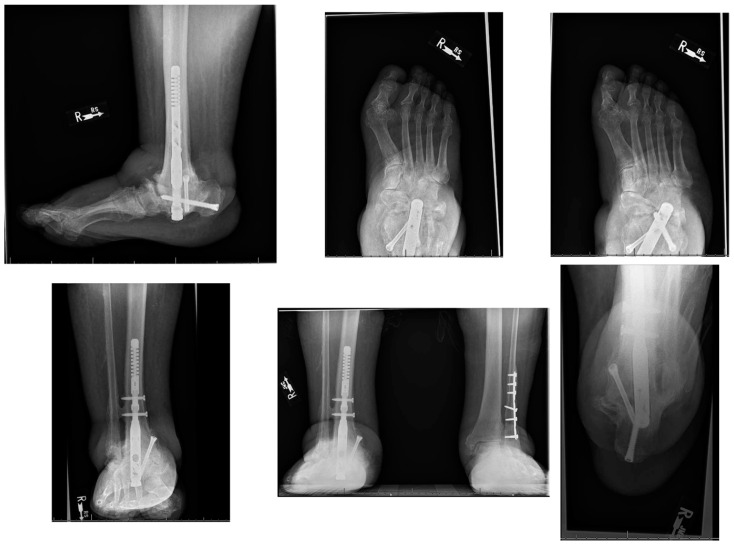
The final one-year postoperative weightbearing radiographs.
